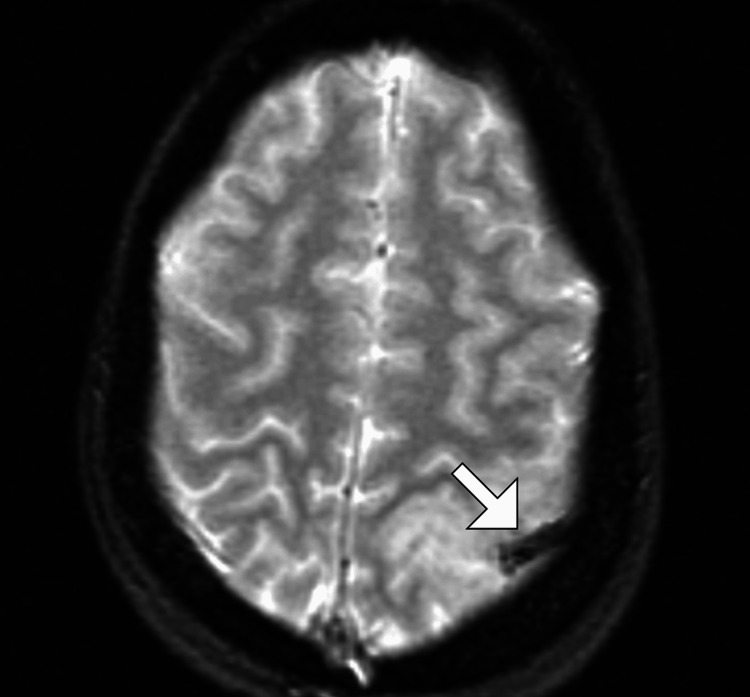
Gradient-echo MRI showing cortical vein thrombosis of the vein of Trolard. Axial gradient-echo MRI demonstrates a blooming artifact (white arrow) in a tubular structure corresponding to the thrombosed vein of Trolard. This blooming is characteristic of deoxygenated blood products within a thrombosed cortical vein and confirms the diagnosis of isolated cortical vein thrombosis.
